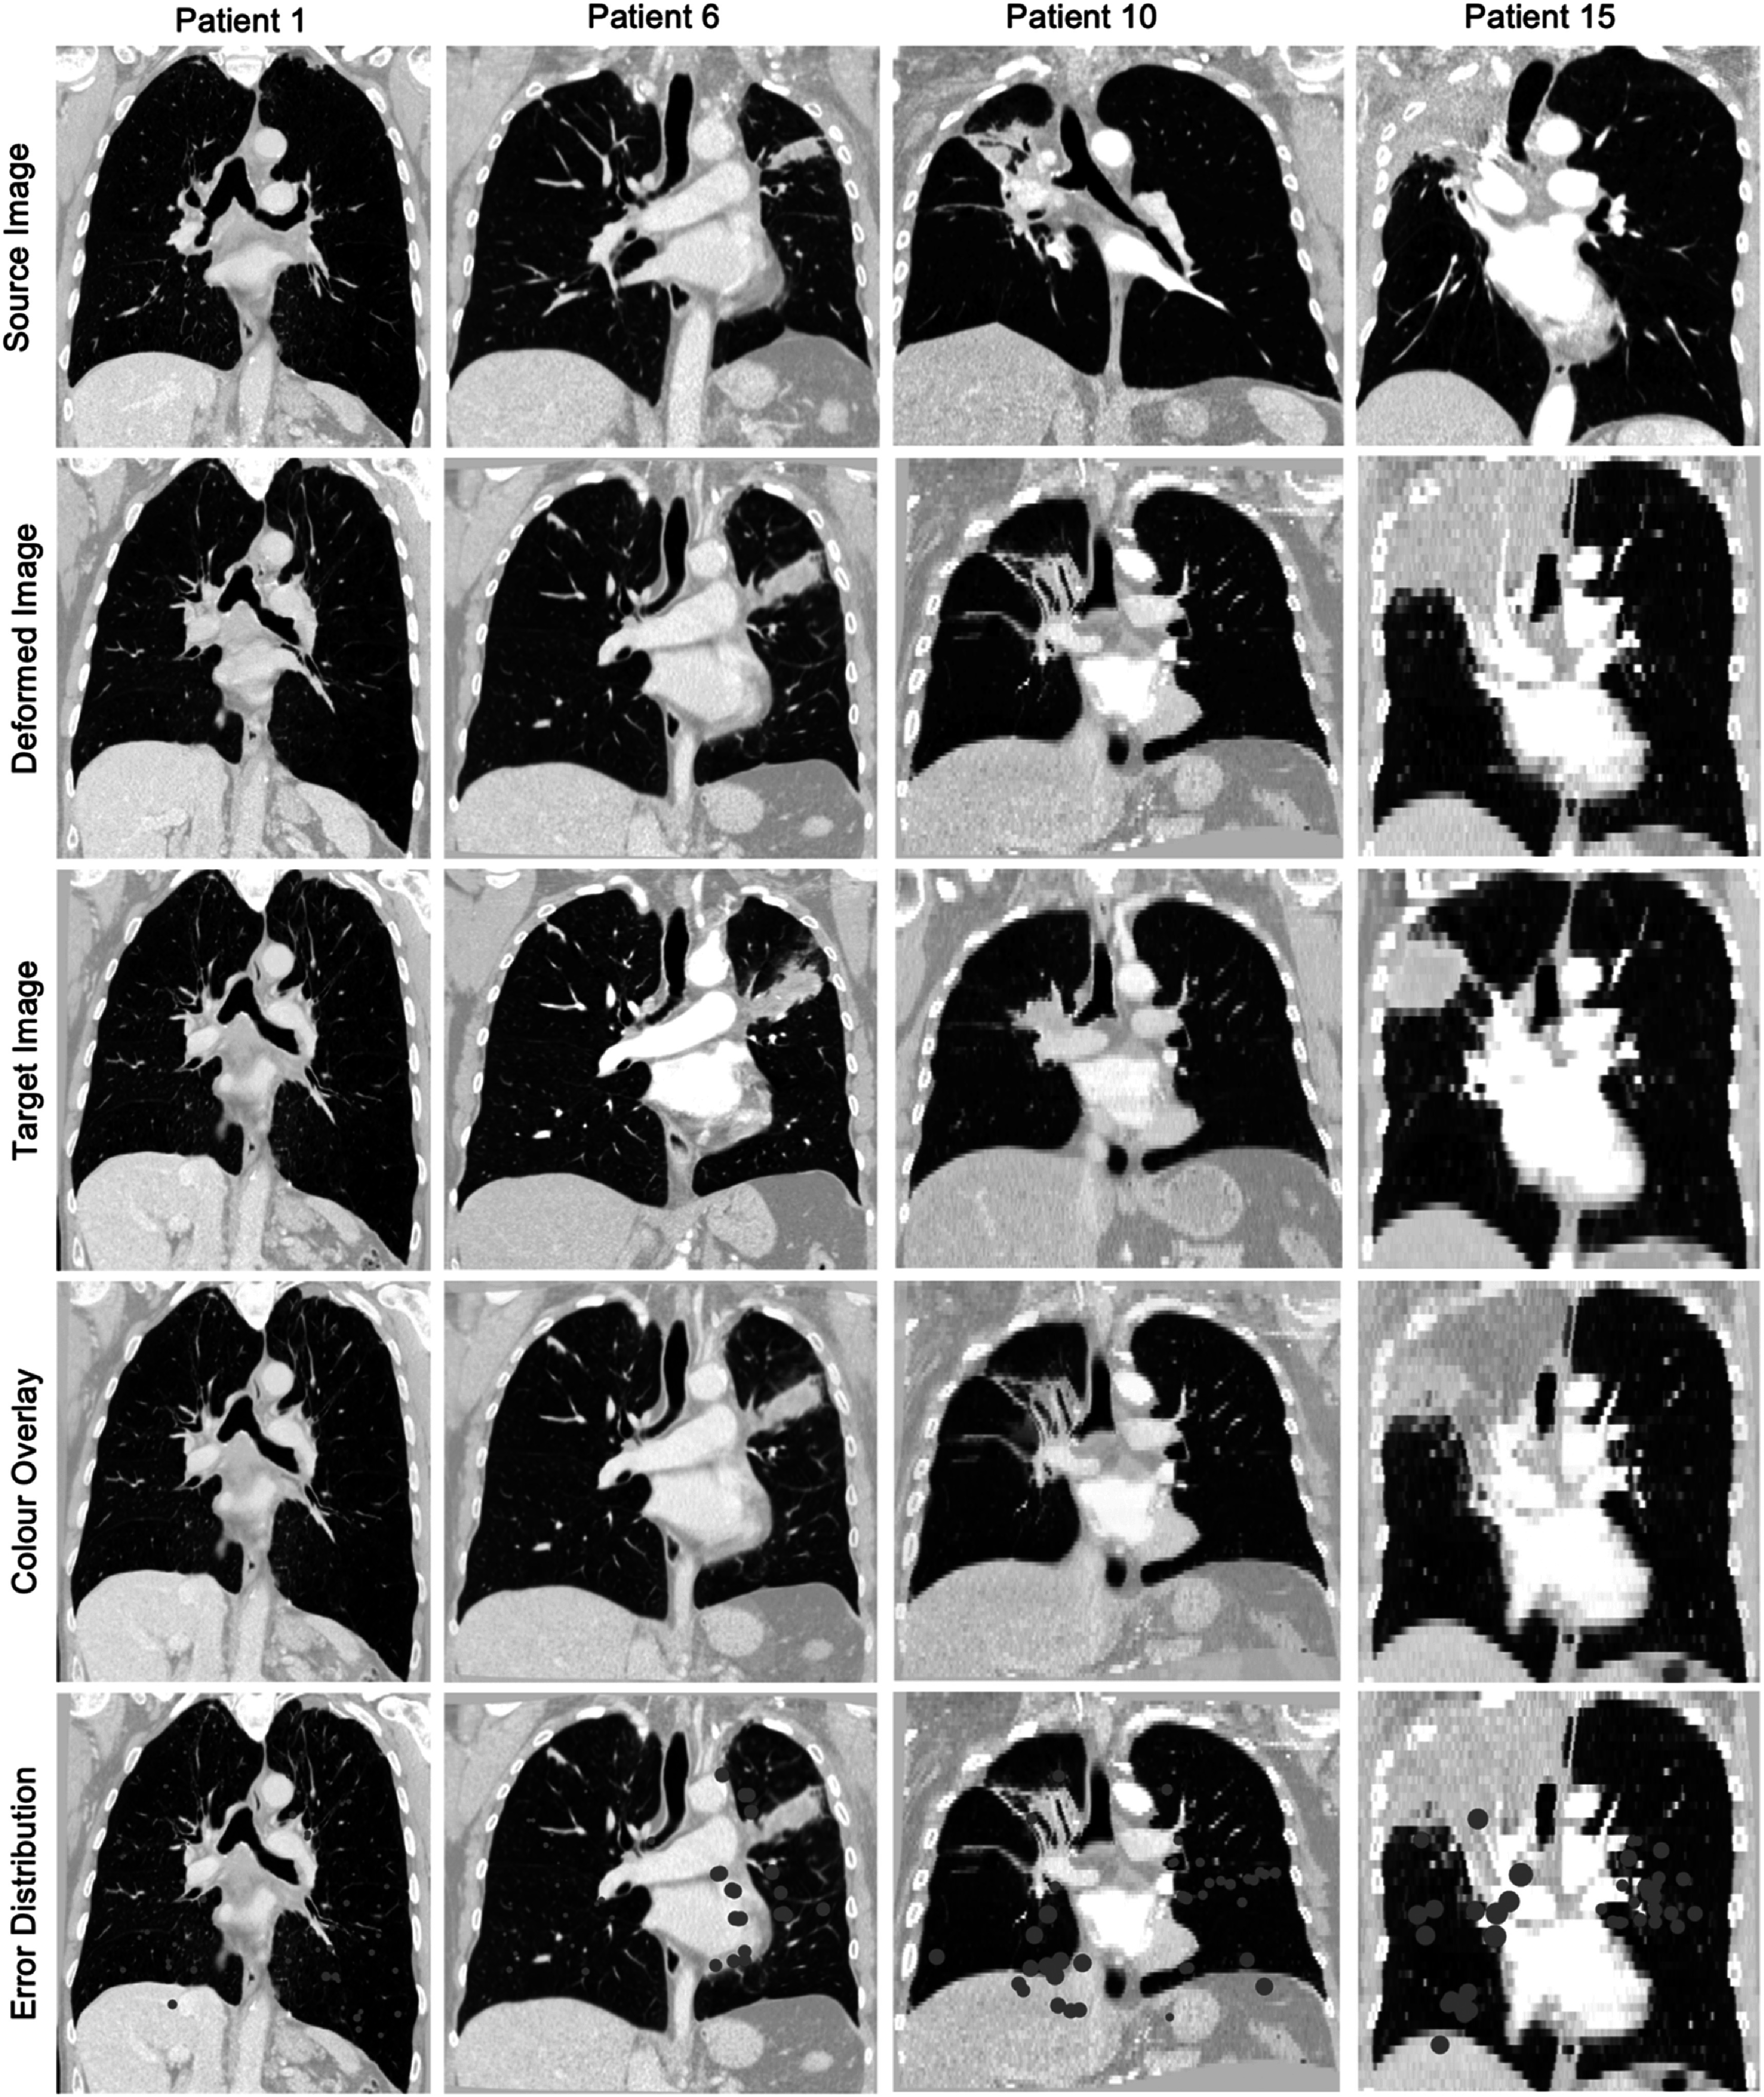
From left to right: example CTs of patients of increasing difficulty. Top to bottom: coronal slices from source CT (12 month follow up), target CT (baseline), deformed CT, colour overlay of target (red) and deformed CT (cyan), landmark points displayed over the deformed CT where the size of the landmark point corresponds to the size of *D*
